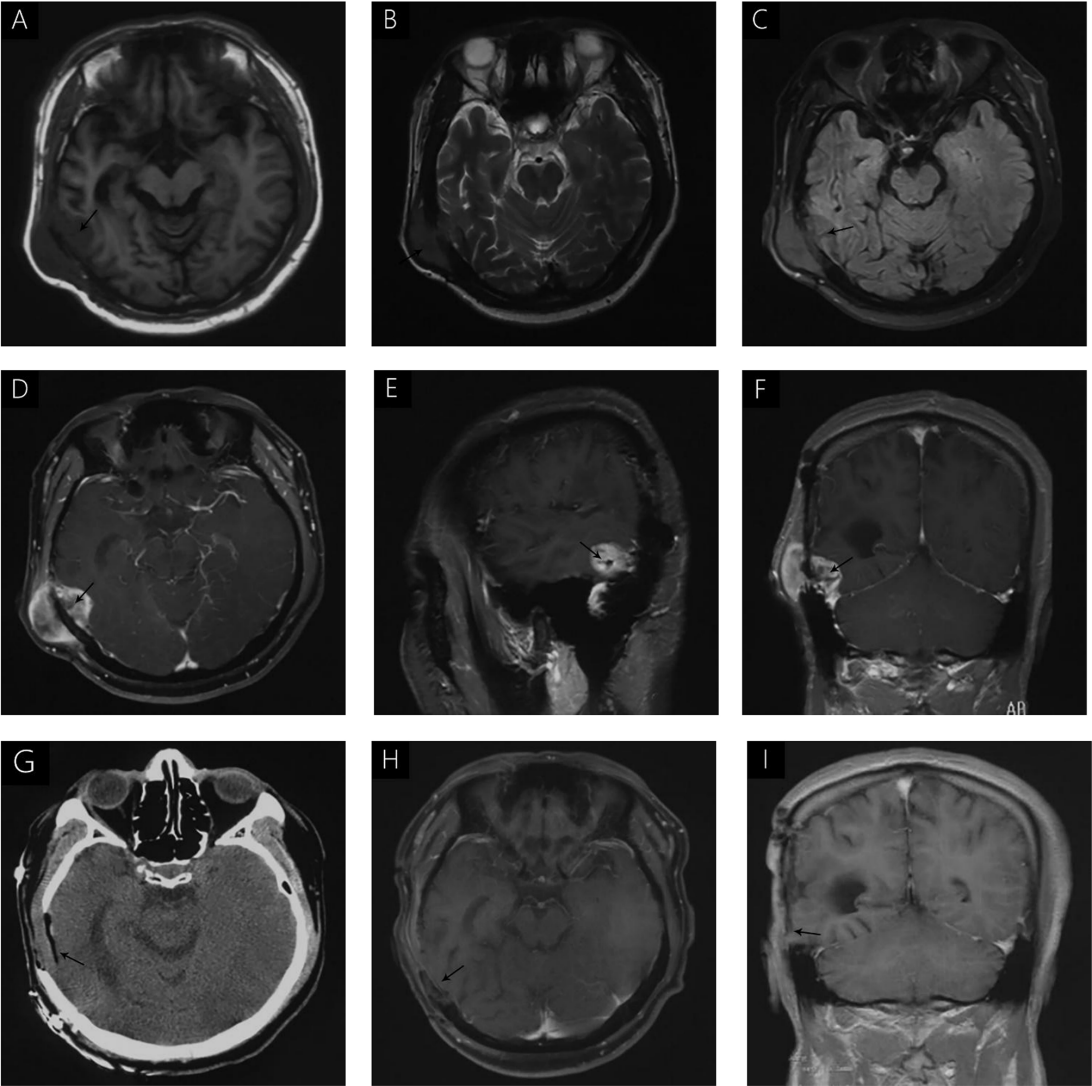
(A) hypointense signal is seen on T1WI (A). Isointense and slightly hyperintense signals were seen on T2WI (B); Isointense signal on FLAIR (C). Significant enhancement was seen after gadolinium administration, without uniform enhancement in the center of the lesion (D–F). Immediate postoperative CT (G) and follow-up MRI, 3 months after surgery (H, I) demonstrated complete removal of the tumor and no signs of recurrence.
